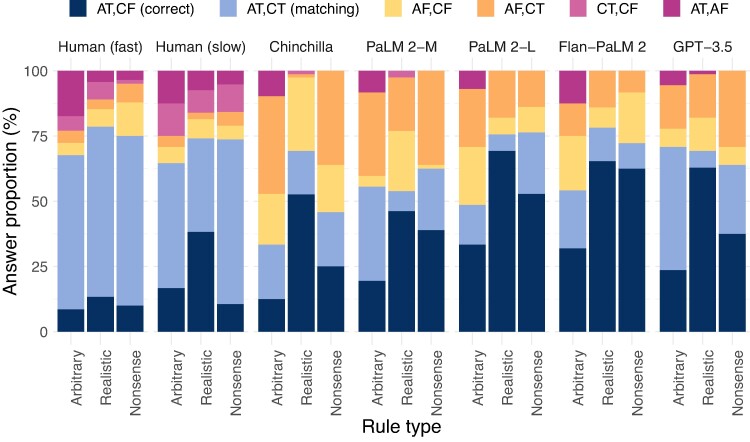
Answer patterns for the Wason tasks, broken down into the pairings of individual cards that each participant chose (AT = Antecedent True, CF = Consequent False, etc.). Behavior is not random, even when performance is near chance. As above, humans do not usually choose the correct answer (AT, CF; dark blue); instead, humans more frequently exhibit the matching bias (AT, CT; light blue). Humans also show other errors, e.g. surprisingly often choosing two cards corresponding to a single rule component (pink). Language models answer correctly more often than humans, but intriguingly choose options with the antecedent false and a consequent card (yellow/orange) more frequently.
